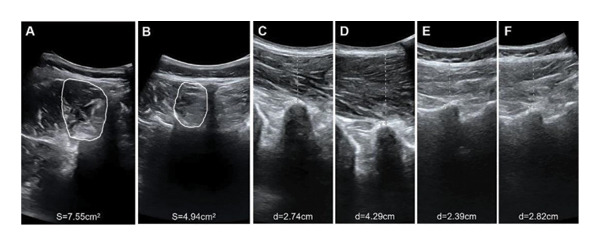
Representative ultrasonographic images of the multifidus muscle from one subject in the HC group and one subject in the CNLBP group. (A) CSA of the multifidus in the HC group; (B) CSA of the multifidus muscle in the CNLBP group; (C) thickness of the multifidus at rest in the HC group; (D) thickness of the multifidus during contraction in the HC group; (E) thickness of the multifidus at rest in the CNLBP group; (F) thickness of the multifidus during contraction in the CNLBP group; HC: healthy control; CNLBP: chronic nonspecific low back pain; CSA: cross‐sectional area.
